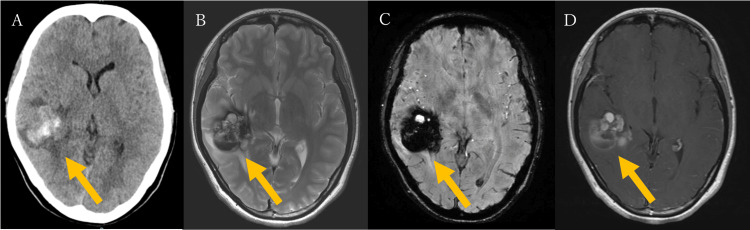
The patient's initial head CT and MRI findings (A) Non-contrast head CT reveals an intracerebral hemorrhage in the right temporal lobe with surrounding brain edema. (B) T2-weighted MRI shows a popcorn-like lesion with a surrounding hypointense rim. (C) Susceptibility-weighted imaging (SWI) demonstrates blooming artifacts around the lesion. (D) Gadolinium-enhanced MRI shows partial contrast enhancement of the lesion.
